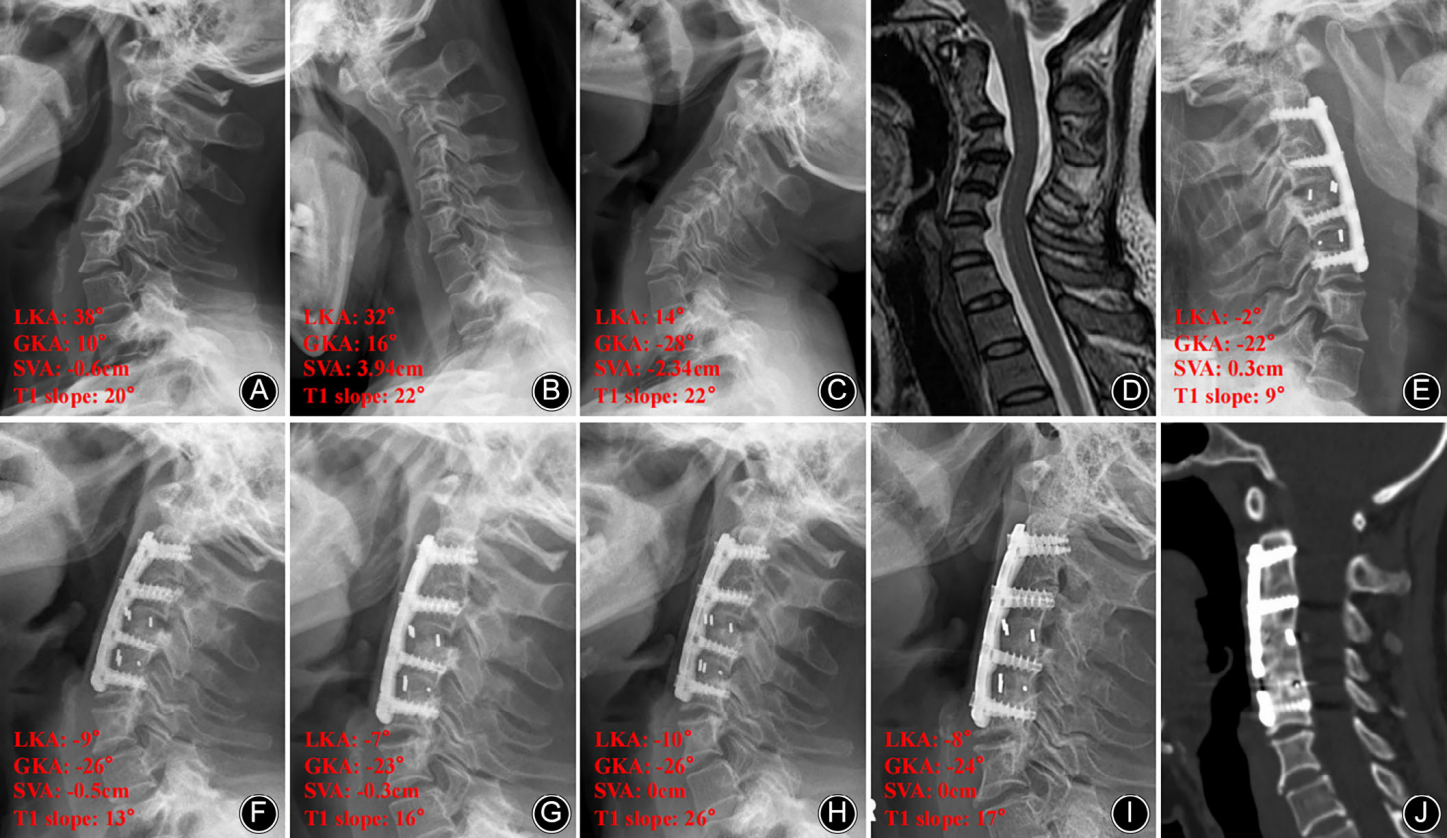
Case 4. A 36‐year‐old woman with Neurofibromatosis‐1 (NF‐1) cervical kyphosis. Preoperative lateral X‐ray (A) showing significant dislocation and scallop of C3 and C4 vertebrae. The local kyphosis angle (LKA) and global kyphosis angle (GKA) were 38° and 10°, respectively. Preoperative flexion–extension X‐ray (B, C) showing flexible cervical kyphosis. The LKA and GKA were 14° and −28°, respectively, in extension X‐ray (C). Preoperative magnetic resonance imaging (MRI) (D) shows compression of the spinal cord at C3/4 level. Postoperative X‐ray (E) showed that the LKA and GKA were −2° and −22° after C3–6 anterior cervical corpectomy and fusion. Kyphosis correction was well maintained at postoperative 12‐month X‐ray (F), 24‐month X‐ray (G), 54‐month X‐ray (H), and 96‐month X‐ray (I), without instrumentation failure (J). At 96‐month follow‐up, the postoperative LKA and GKA reduced to −8° and −24°, respectively (I, J).
